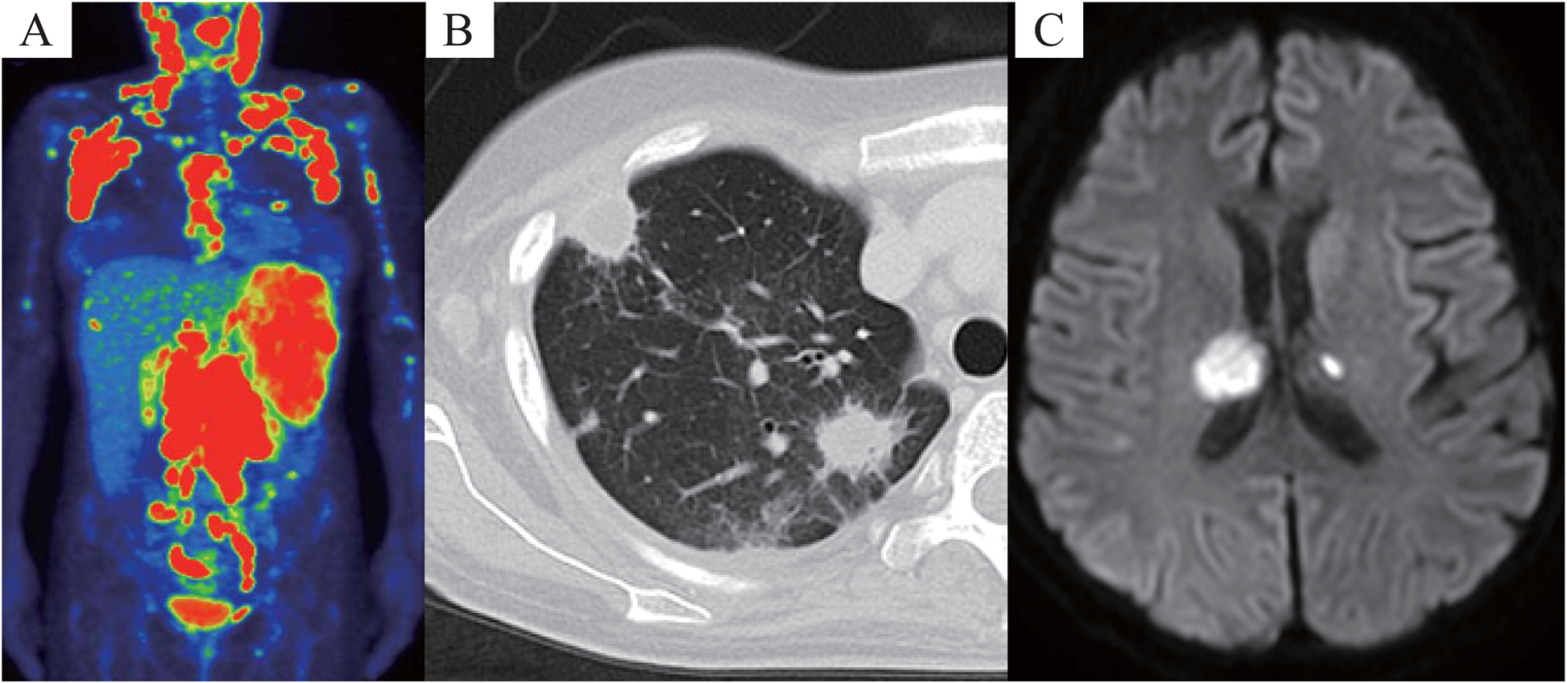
Scans obtained from ^18^F-fluorodeoxyglucose positron emission tomography-computed tomography (a), chest computed tomography (b), and head magnetic resonance imaging (c). An increase in the uptake of fluorodeoxyglucose in the cervical, axillary, mediastinal, and mesenteric lymph nodes, spleen, and bone marrow was observed 42 days postpartum (a). Multiple nodular shadows and pleural effusion were observed in both fields 9 months postpartum (b). Diffusion weighted image axial brain magnetic resonance imaging showing the evolution of cerebral infarction 9 months postpartum (c)
